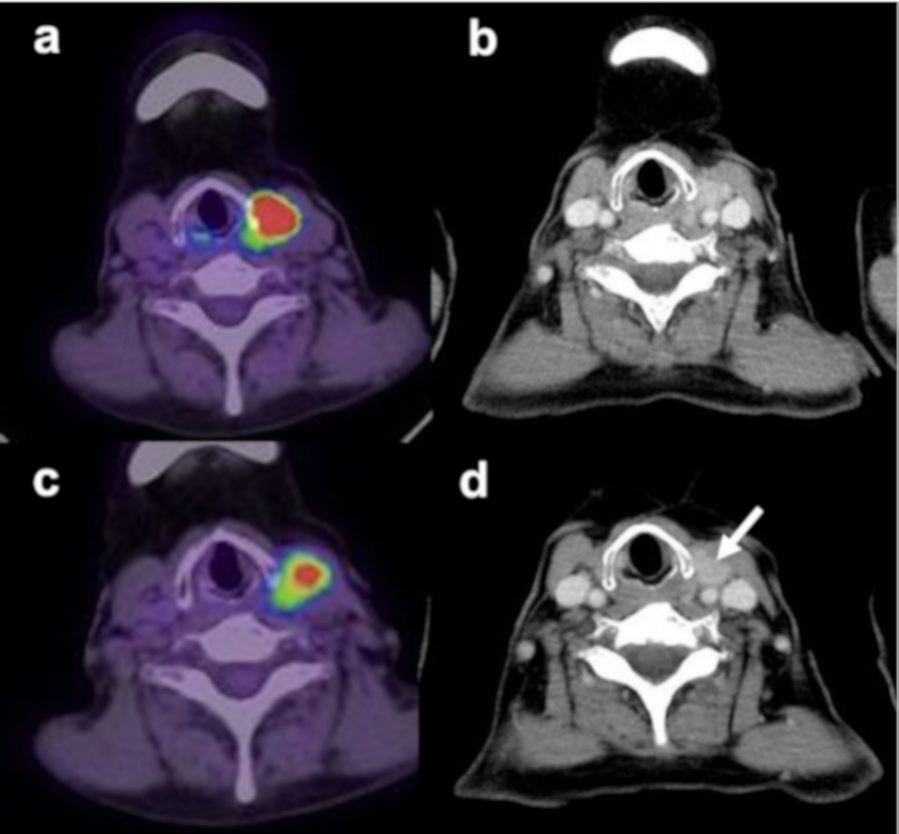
Imaging findings in 67-year-old patient with papillary carcinoma undergoing PET/CT and contrast-enhanced CT. At the initial treatment, PET/CT (a) and contrast-enhanced (b) showed a 2 cm primary tumour in the left thyroid with an SUVmax of 48.7. Systemic chemotherapy with a lenvatinib dose of 24 mg was initiated as the first-line therapy. After 1 week of treatment with lenvatinib, PET/CT (c) demonstrated a remarkable decline in SUVmax to 24.2. However, the mass is still visualised on contrast-enhanced CT (d) performed 4 weeks after treatment (arrow). At the time of writing this report, the patient was alive for more than 6 years after treatment. SUVmax: maximum standardised uptake value
